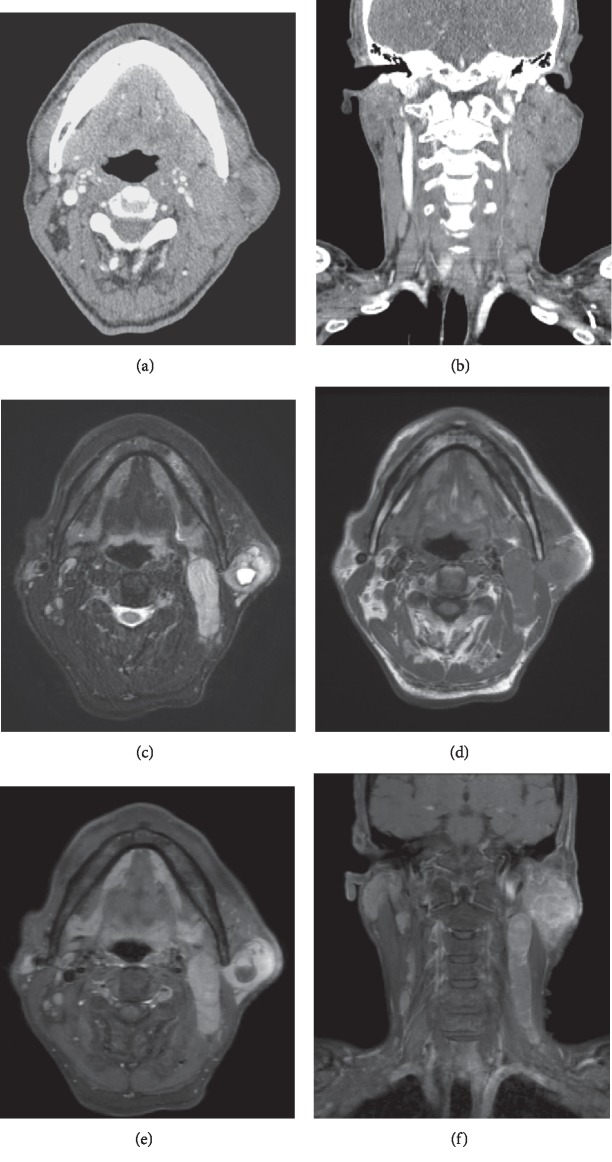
(a, b) A CT scan demonstrated a nodular, isodense, and necrotic mass in the left parotid gland and multiple homogenous density, necrotic, and enlarged lymph nodes in the left lateral neck. (a) Postcontrast axial CT image and (b) coronal image. (c–f) MRI of the parotid gland and neck revealed a lobulated necrotic mass that involved the superficial and deep lobes of the left parotid gland and multiple homogenous densities with necrotic enlarged lymph nodes in the left lateral neck. Indeterminate enlarged lymph nodes were observed on the right lateral neck. (c) T2-weighted axial image, (d) T1-weighted axial image, (e) postcontrast T1-weighted axial image, and (f) postcontrast T1-weighted coronal image.
